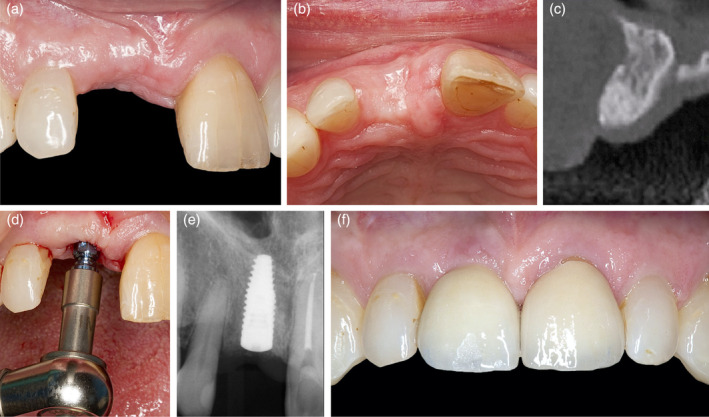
(a) and (b) Clinical and (c) radiographical situation after a healing period of 9 months prior to implant installation (d) and (e) and prosthetic restoration (f)
